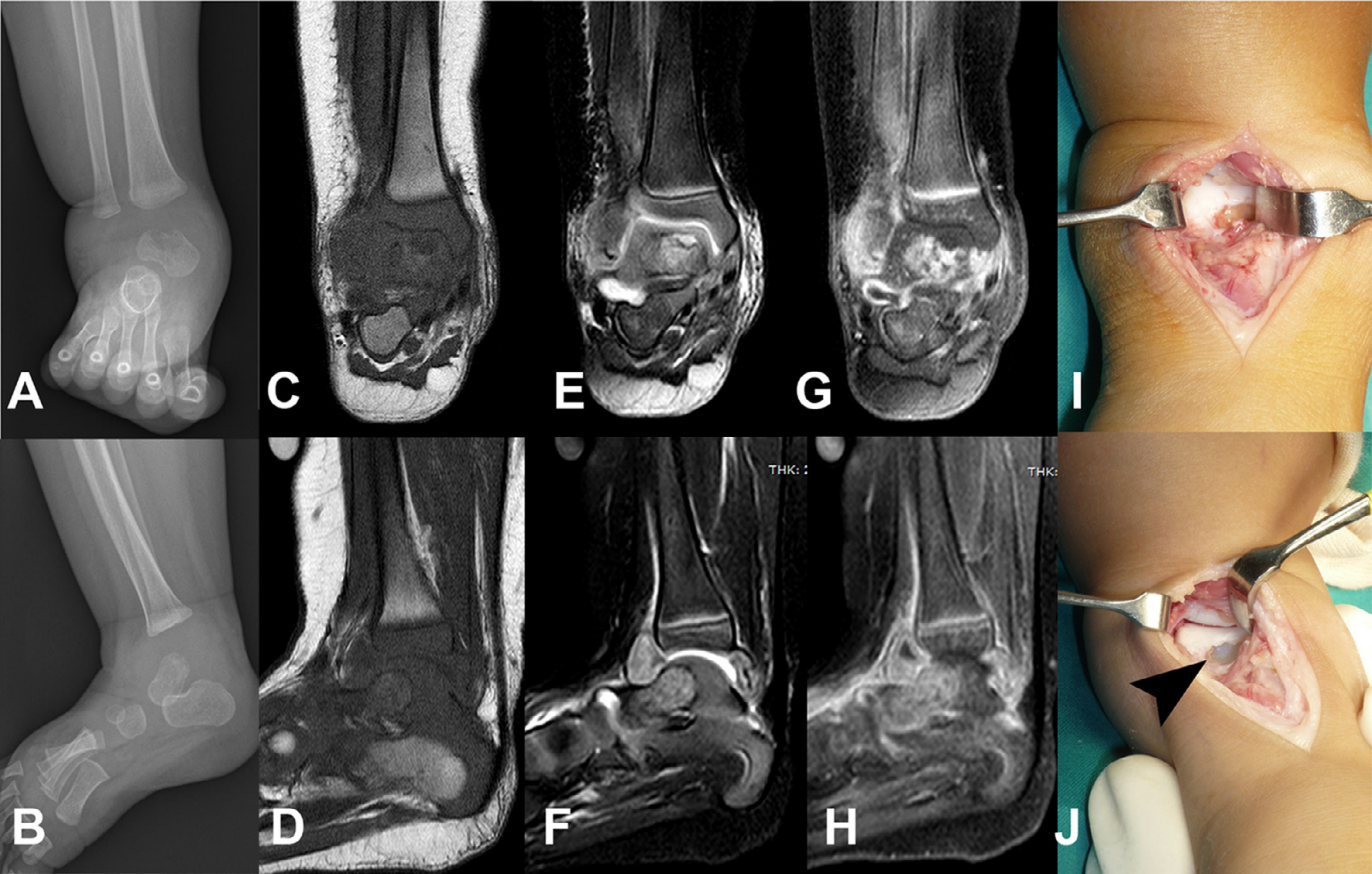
Anteroposterior (A) and lateral (B) radiographs of the right ankle of patient 1 are shown along with magnetic resonance imaging images in T1 weighted (C, D), T2 weighted (E, F), and gadolinium-enhanced (G, H). Intraoperative finding before (I) and after (J) debridement is demonstrated. The arrowhead represents an osteolytic lesion at the talus.
